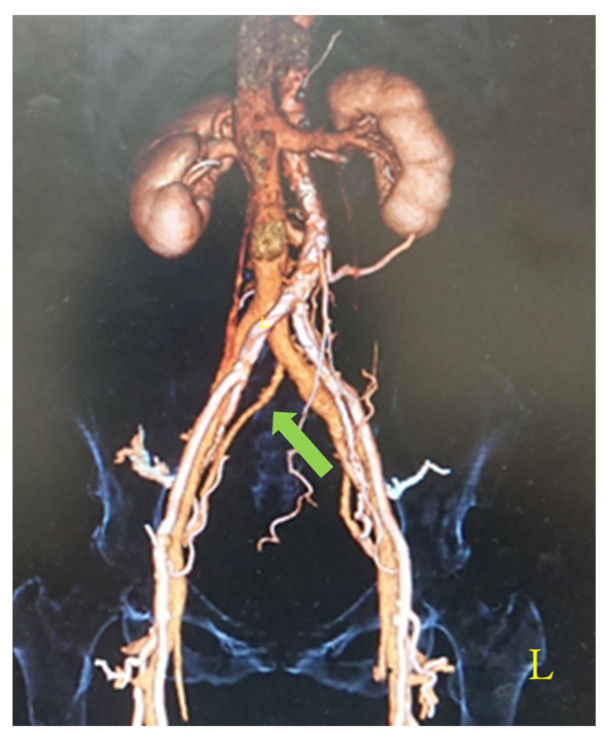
Representative contrast-enhanced CT image showing anomalous drainage of the unilateral internal iliac vein into the contralateral common iliac vein. Arrows indicate the anomalous venous course. This image represents a typical case observed in this study.
